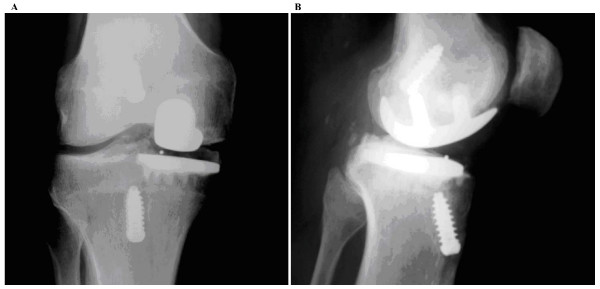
Radiograph of unicompartmental replacement with ACL reconstruction.
